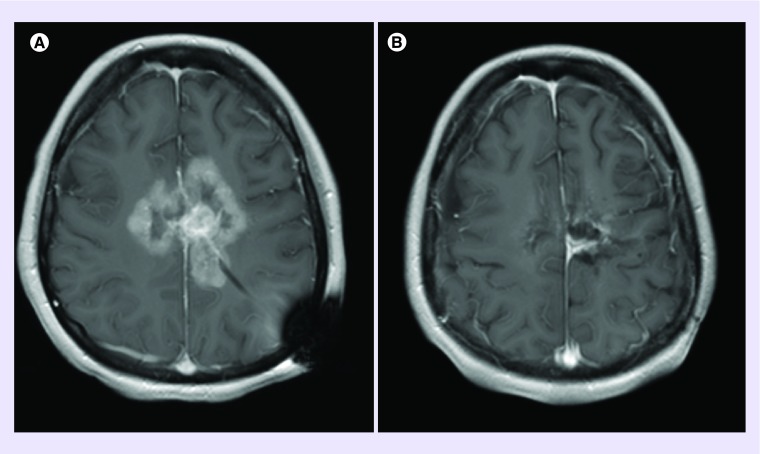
MRI prior to and following treatment with BRAF inhibitor and concurrent tumor treating field. Axial T1 post-contrast MRI images from (A) 7 February 2017, and (B) 8 August 2017.
